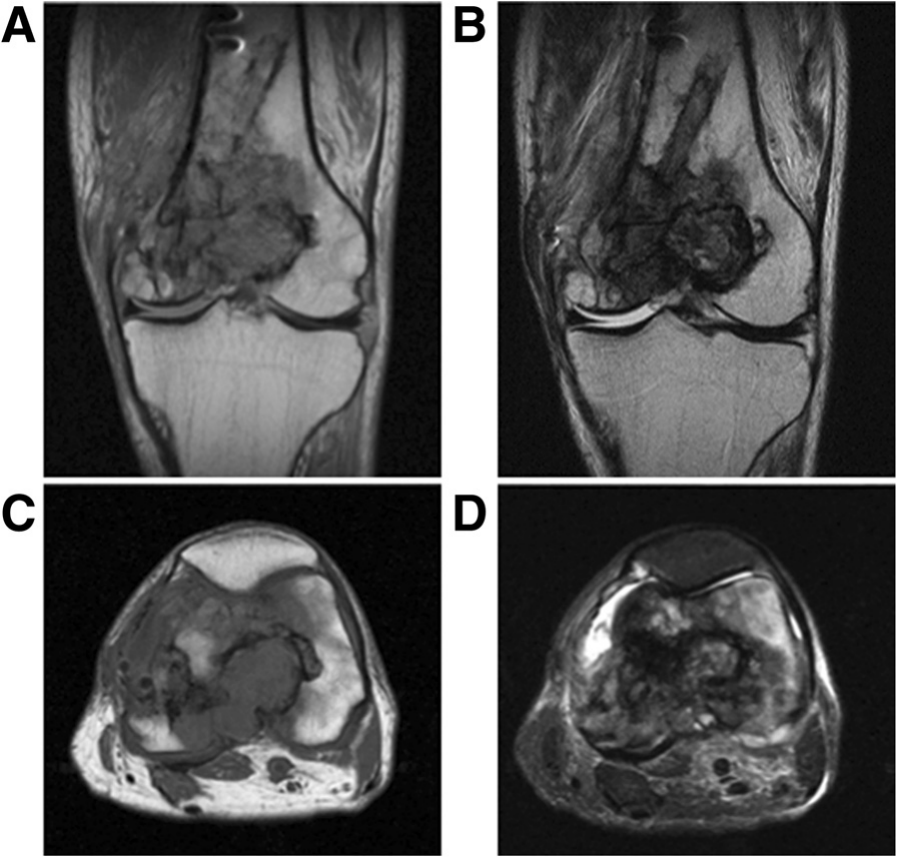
Magnetic resonance imaging. Coronal section: a T1-weighted image, b T2-weighted image. Transverse section: c T1-weighted image, d short T1 inversion recovery. Fluid retention can be seen in the medial femur and intra-articular area. T1-weighted images of the intra-osseous area show a low to isosignal intensity, and T2-weighted images showed an isosignal to high signal intensity. There are no masses in the extra-osseous area
